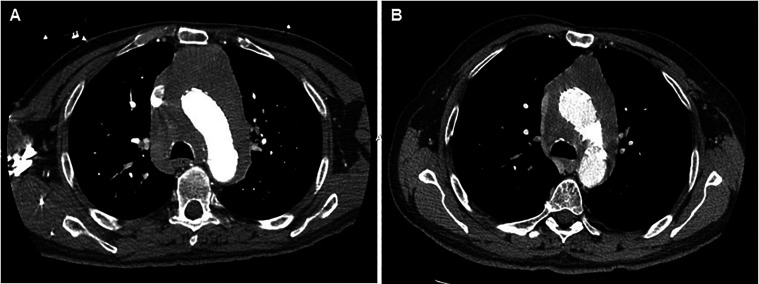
Ct angiogram of the chest showed a soft tissue infiltrative mass in the mediastinum replacing the mediastinal fat and encasing the aortic arch (A). The largest diameters at the level of the aortic arch were 11.0 × 10.0 cm. CT of the chest 1 month after treatment with steroids showed interval improvement in the size of the infiltrative lesion extending from the level of the arch vessels down to the aortic root with the largest diameters at the level of the aortic arch are 10.0 × 7.7 cm (B).
